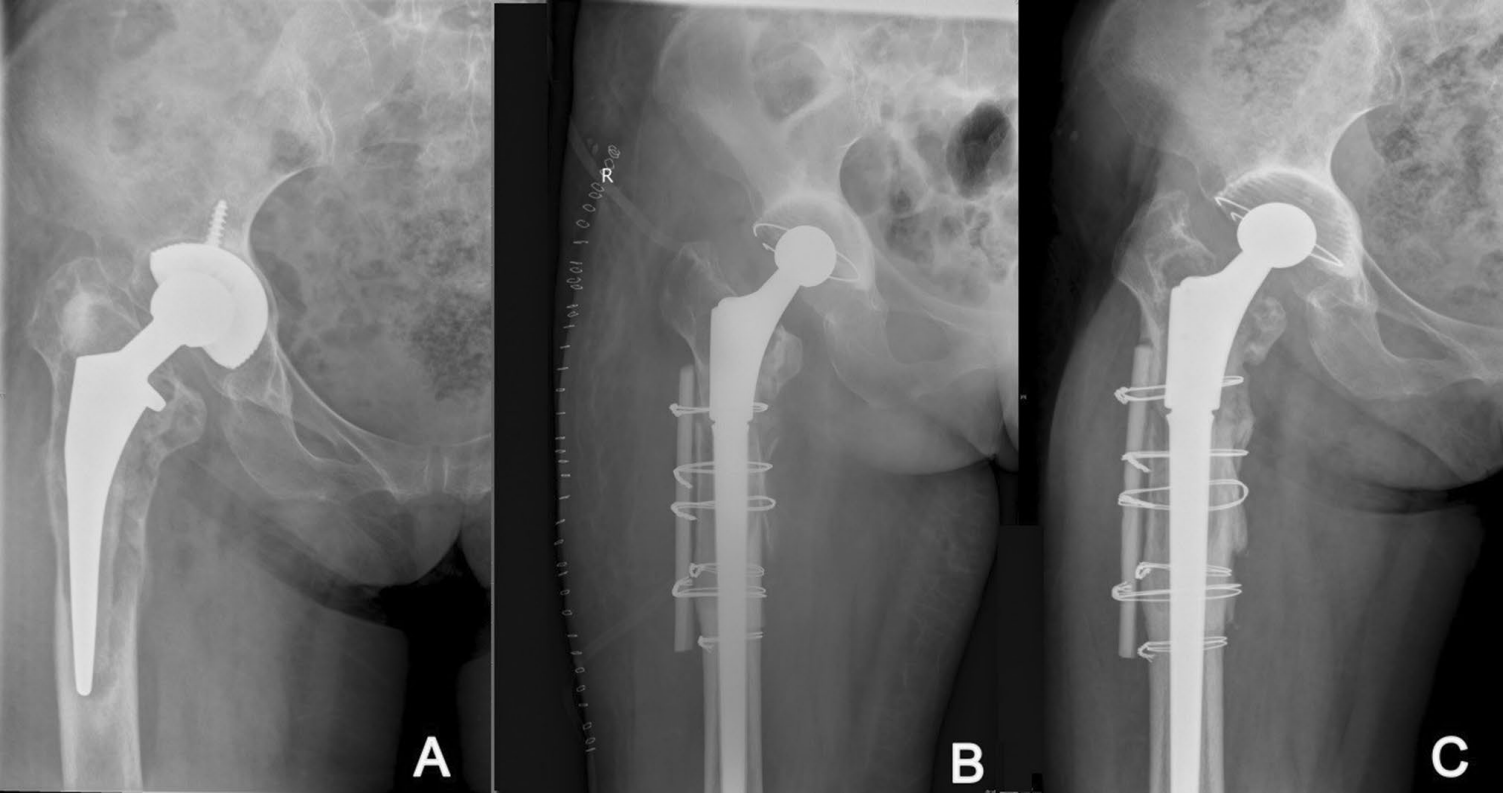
Preoperative and postoperative radiographs of high-grade femoral defect managed with a modular stem with failed osseointegration and stem subsidence.
